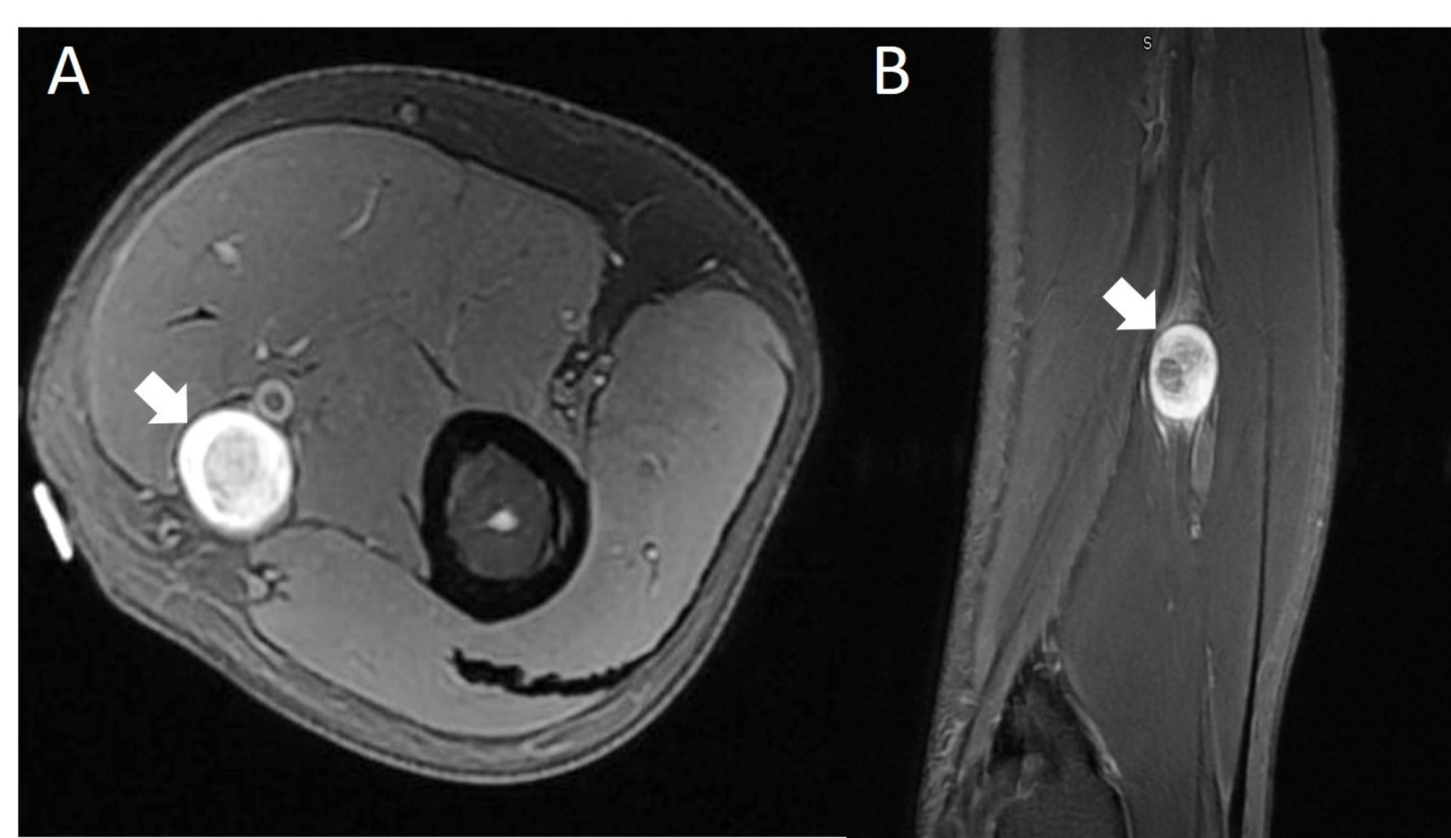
Magnetic resonance imaging (MRI) of the left upper extremity (A) Axial T1 MRI with contrast demonstrating spherical mass in the proximal anteromedial upper arm. (B) Sagittal T1 MRI with contrast demonstrating continuity with the median nerve.
